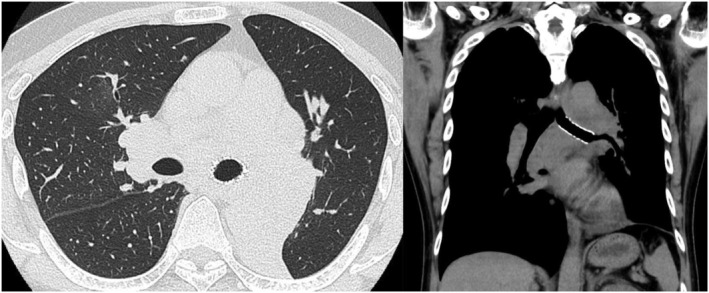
CT finding after AERO stent placement.
